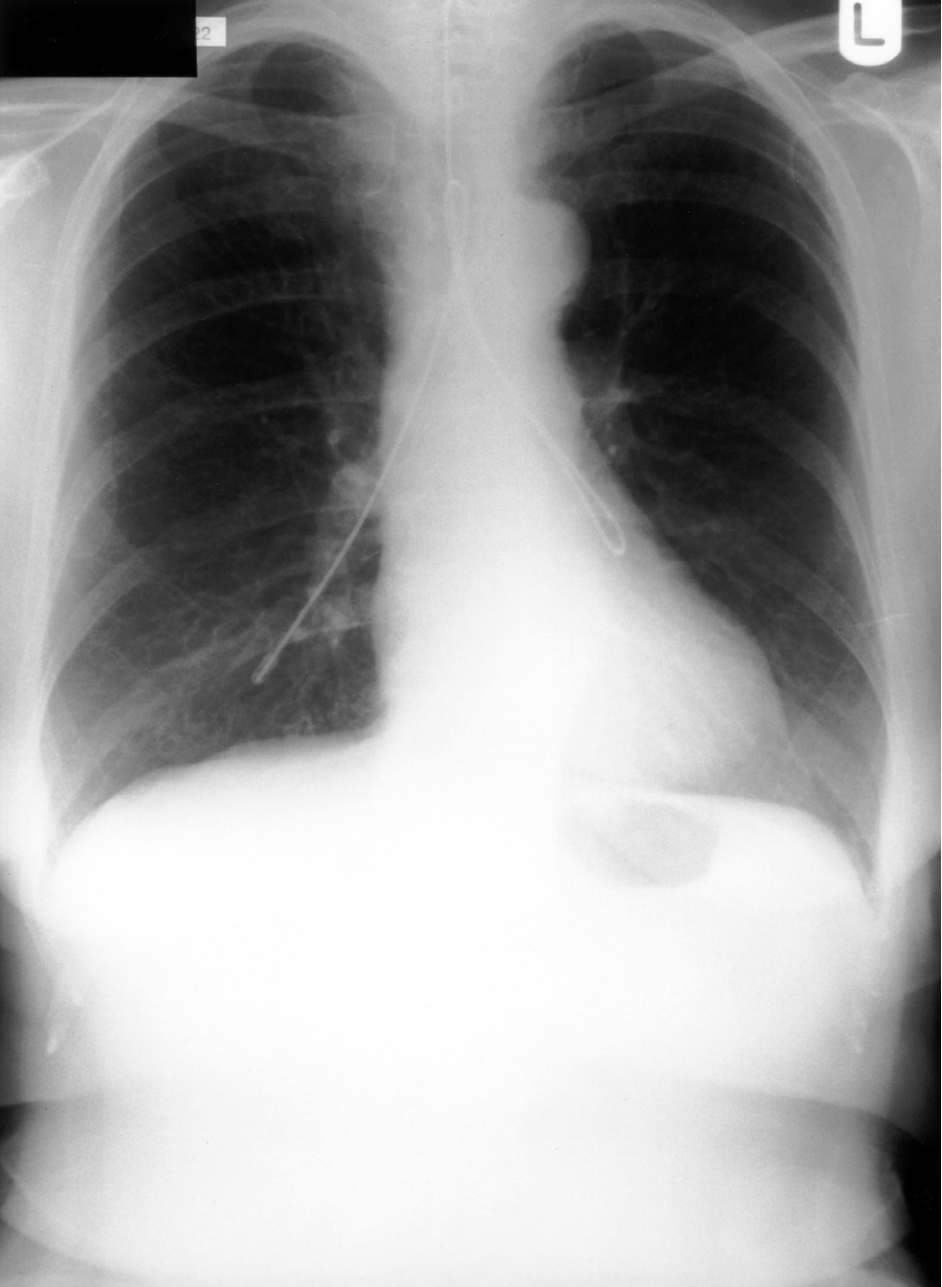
Chest x-ray showing a nasogastric tube folded into both main bronchi of a patient.
